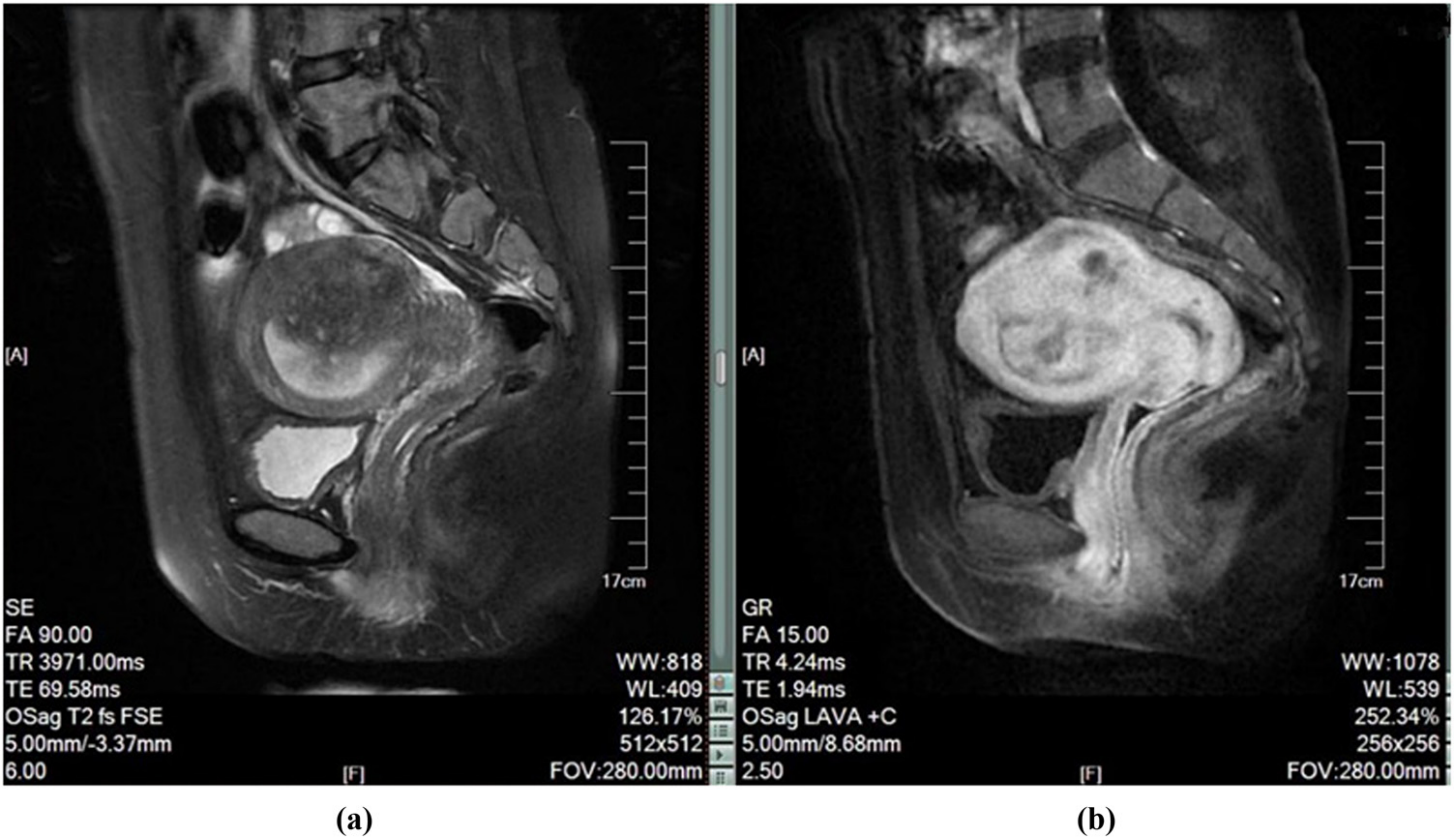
MRI image before treatment: (a) T2 sagittal MRI image and (b) enhanced sagittal MRI image.
